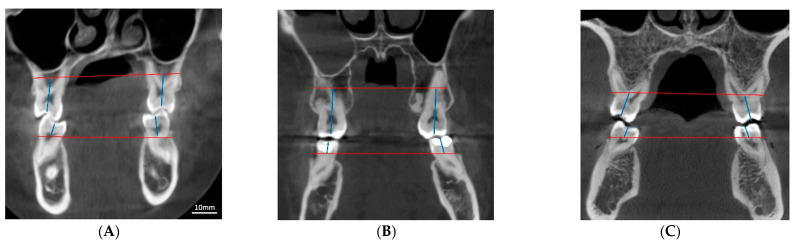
Example of different combinations of arch width and molar inclination. (A): Normal maxillary width and normal molar B-L axial inclination. (B): Constricted maxilla, normal molar B-L axial inclination, presence of bilateral posterior crossbite. (C): Constricted maxilla, buccally flared maxillary molars, absence of posterior crossbite. (The red lines in the CBCT images indicate the measured widths of the maxilla and mandible. The blue lines represent the inclinations of the molars on the CBCT images).
